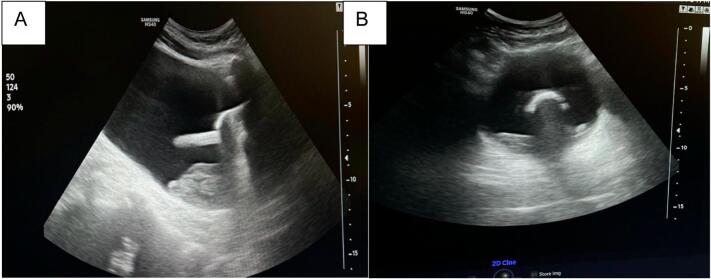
(A,B)- Ultrasonography of bladder in showing tip of foley catheter sitting over the debris with balloon inflated with air and water.
